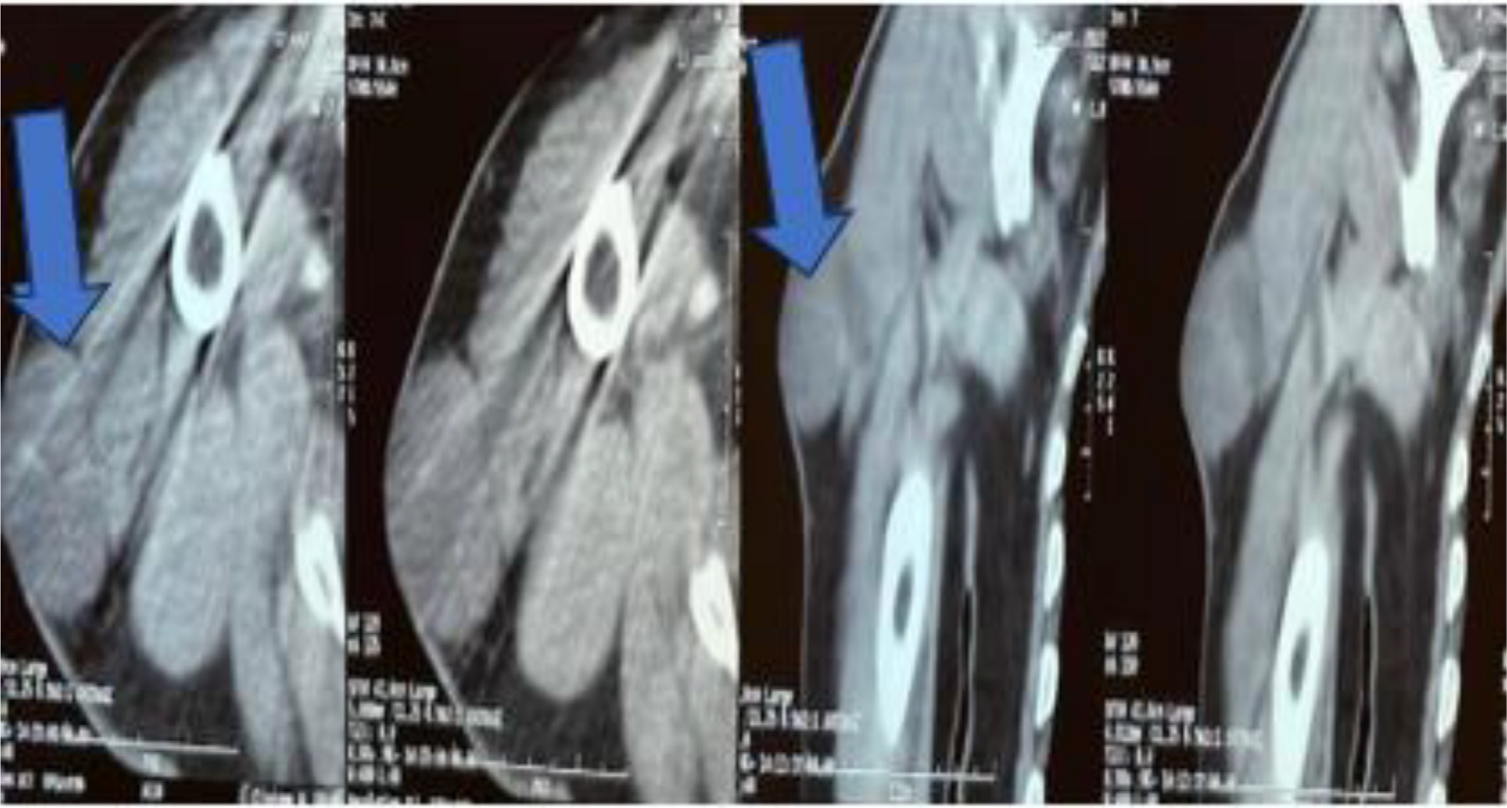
Showing CT scan of the arm revealing a subcutaneous mass in the upper third of the outer aspect of the right arm, well-defined oval in shape, with regular contours, heterodense on contrast, containing a large liquid area and a fleshy component enhanced after contrast. This mass infiltrates the skin covering and presents intimate contact with the deltoid muscle with persistence of a fatty separation border.
